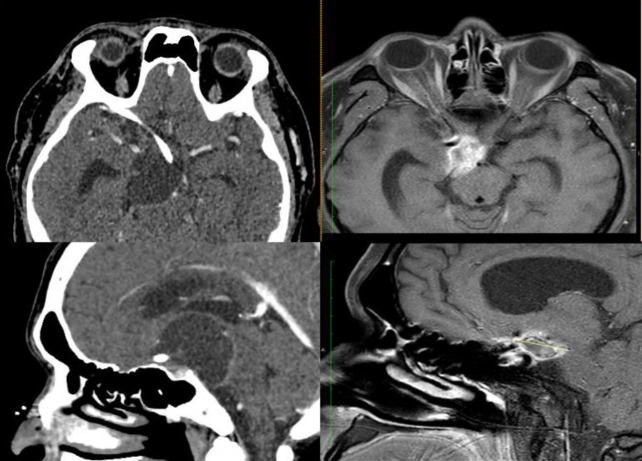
A. Pre therapy CECT (06/2023); B. Post therapy contrasted MRI (06/2024). Post therapy MRI demonstrated smaller suprasellar lesion with less mass effect onto the surrounding structures
